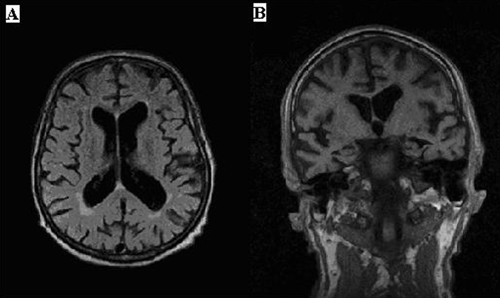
The brain MRI of patient A1. Axial (A) and coronal (B) MRI images of the brain of patient A1 demonstrate moderate central and cortical atrophy.
